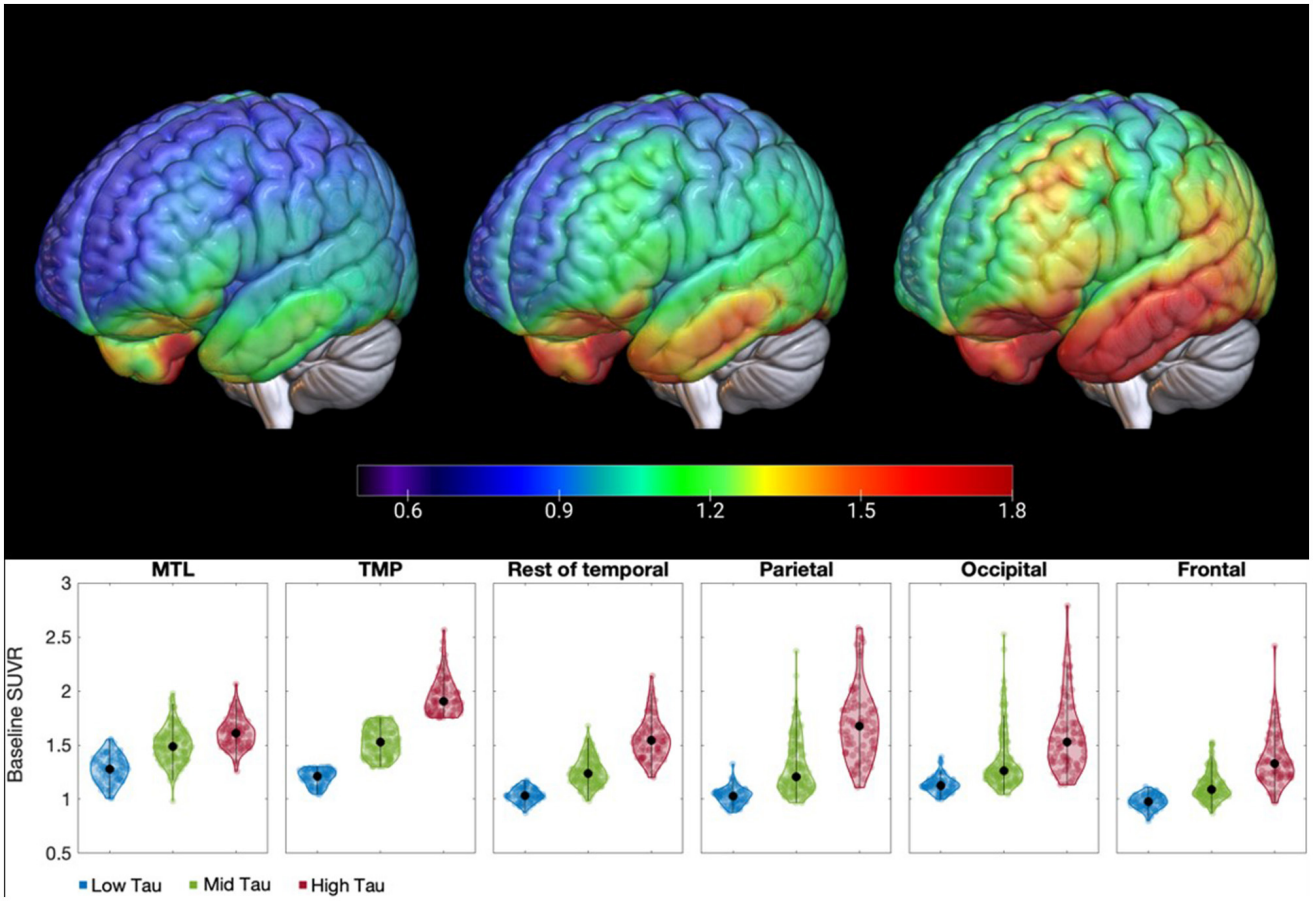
Surface maps and violin plots of [^18^F]GTP1 SUVR displaying the distribution of tau burden by tau level at baseline in the Tauriel study in prodromal and mild AD participants: low tau (TMP SUVR ≤1.30), mid tau (1.30 < TMP SUVR ≤ 1.75) and high tau (TMP SUVR >1.75). The pattern of the uptake from low to high tau burden in the temporal meta-regions of interest is maintained across the cortex. AD, Alzheimer's disease; MT, mesial temporal regions; SUVR, standardized uptake value ratio; TMP, temporal meta-regions of interest.
